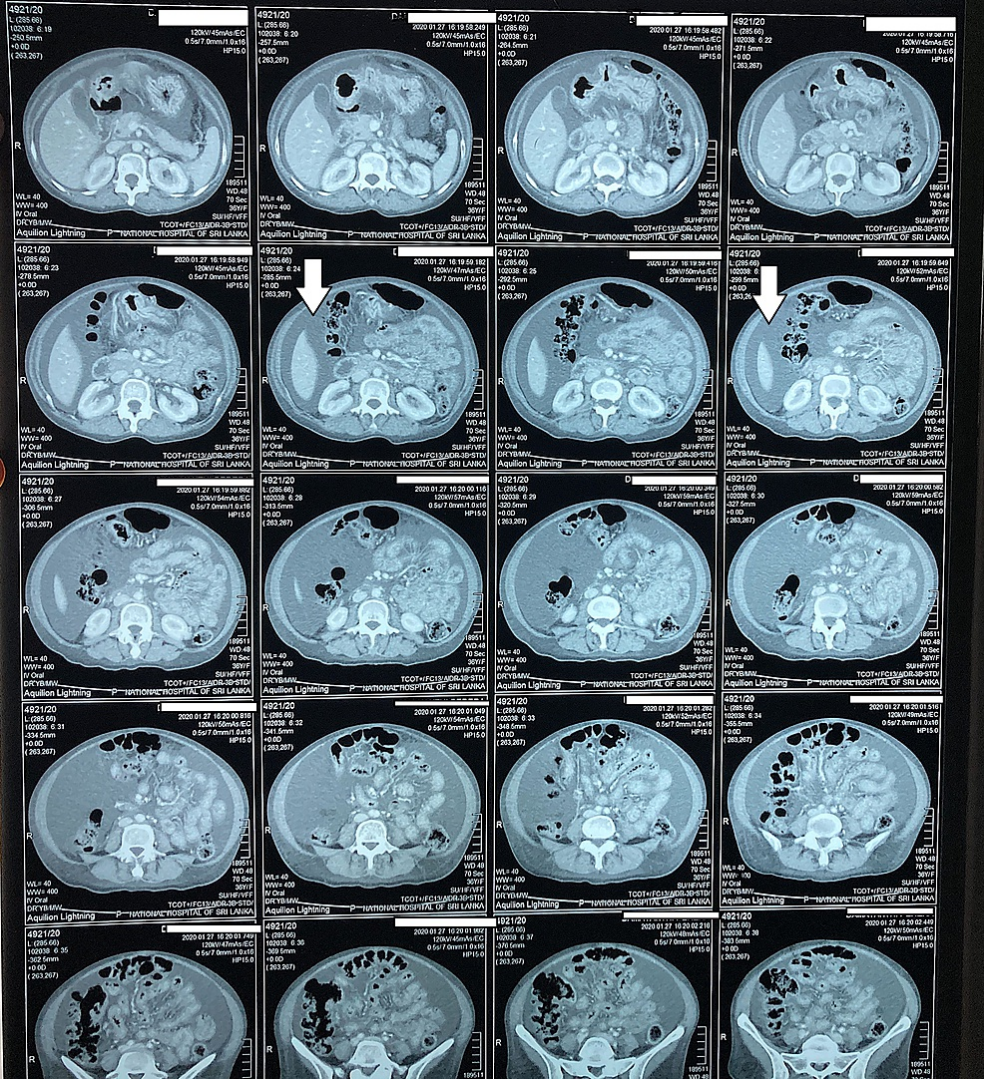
CT chest, abdomen and pelvis shows gross ascites.
